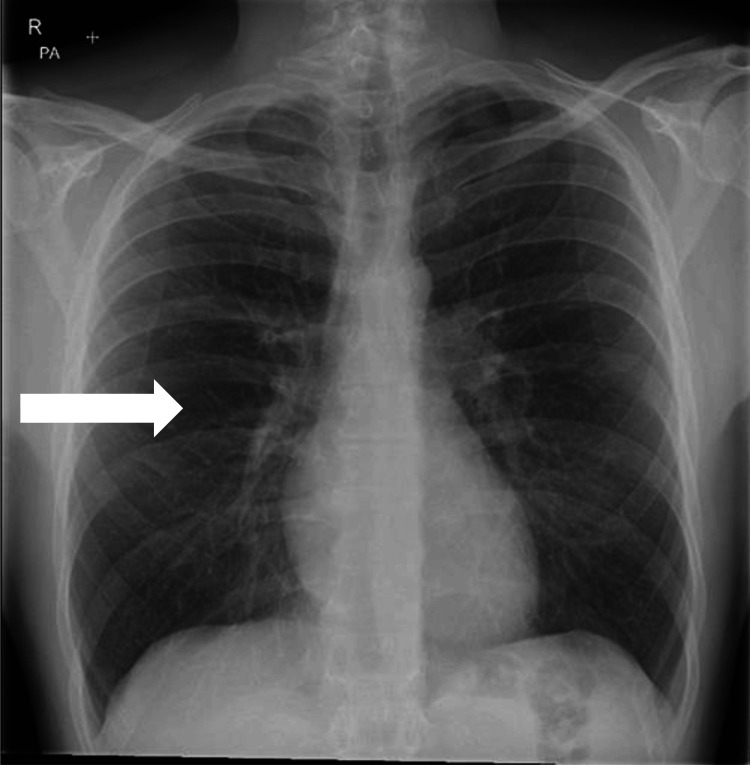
Chest X-ray of Patient Taken on Admission Showing Lung Hyperinflation This chest X-ray shows hyperinflation. There are more than six ribs visible anteriorly. The arrow indicates widened intercostal spaces, also typical of lung hyperinflation in COPD. No acute pathology can be appreciated. COPD: chronic obstructive pulmonary disorder
